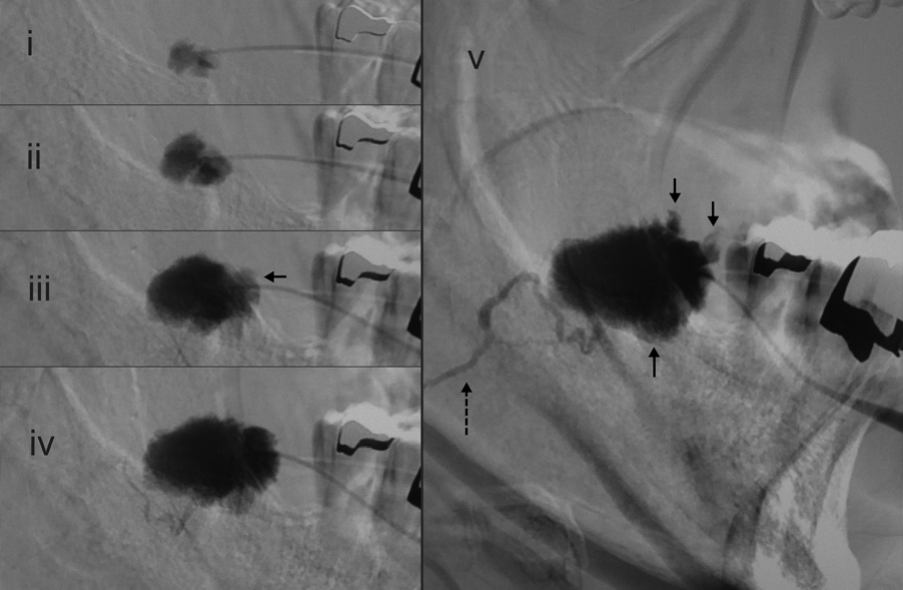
Serial digital subtraction images during continuous injection of a cheek mass that swelled during mastication, in a middle-aged woman. Serial filling of the cisterns is observed (a–c). Draining veins appeared after filling of the cisterns (Type 2B). Unsubtracted images (d–f) obtained during pauses in injection show that the draining veins disappeared within seconds but the cisterns remained filled, showing Type 1 appearance. Plain CT images show calcification in the lesions in the left masseter muscle (g) and sustained pooling inside the fascia of the masseter muscle 4 hours after injection (h). Symbols: black arrow, newly emerged cistern; dotted arrow, draining vein
